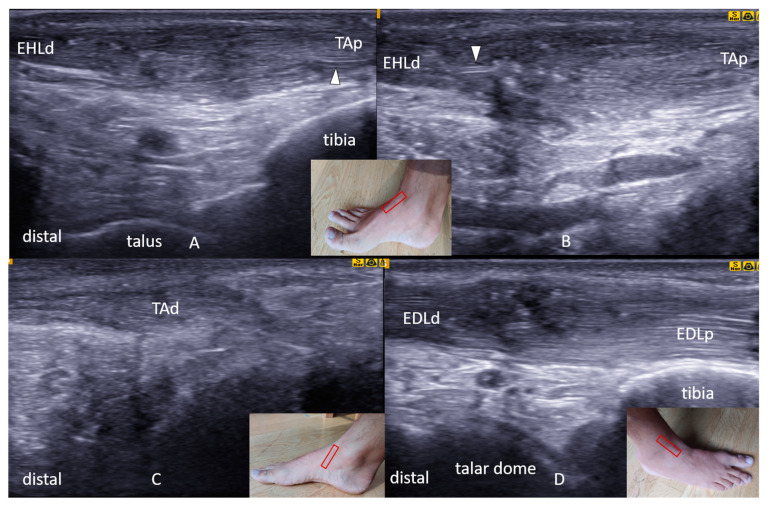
(A,B) Longitudinal ultrasound imaging of the dorsal aspect of the left ankle showed that the EHLd has clear continuity with the TAp. The junction is hypoechoic, thickened, and disorganized with visible suture material (white arrowheads). (C) Longitudinal ultrasound imaging of the anteromedial aspect of the ankle showed that the TAd stump ends blindly, attached to the subcutaneous tissue proximally. (D) The extensor digitorum longus tendon evaluation showed a normal anatomic course of the proximal and distal parts of the sutured tendons. The insets show the transducer positions (red rectangles) during scanning. EHLd, extensor hallucis longus distal end; TAp, tibialis anterior proximal end; TAd, tibialis anterior distal end; EDLp, extensor digitorum longus proximal end; EDLd, extensor digitorum longus distal end; EHLp, extensor hallucis longus proximal end; TA, tibialis anterior tendon; EHL, extensor hallucis longus.
